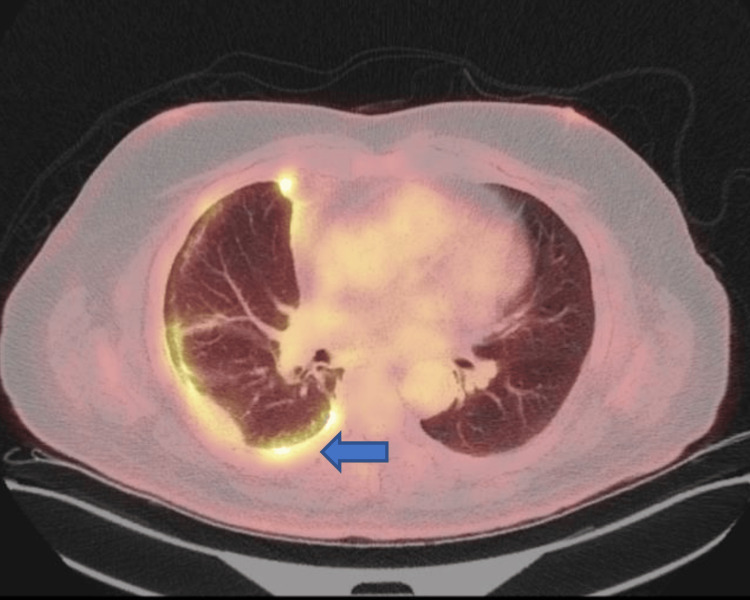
PET scan showing increased FDG activity in multiple foci throughout the right pleura, mostly posteriorly (arrow) PET: positron emission tomography; FDG: fluorodeoxyglucose
